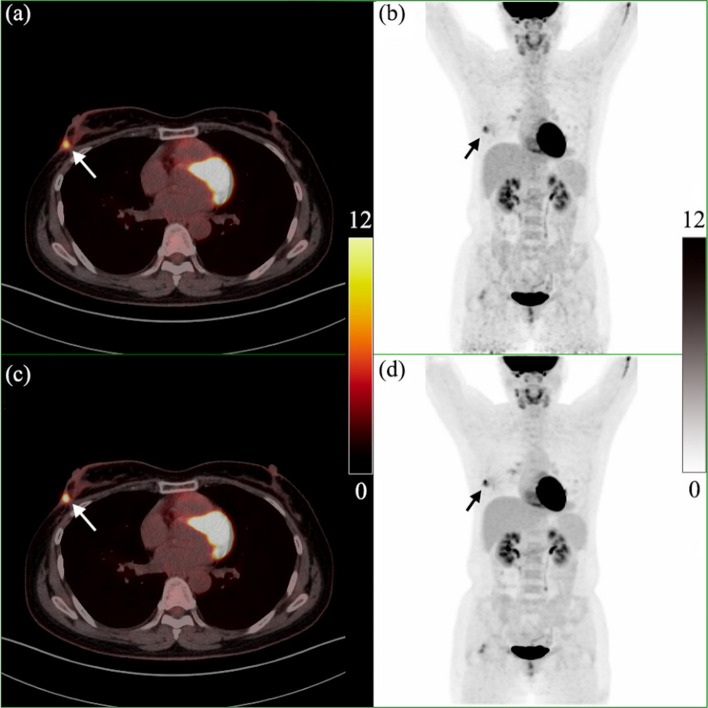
PET/CT image of a breast cancer patient. a and c are PET/CT fusion images reconstructed by OSEM and DPL, respectively; b and d are MIPs reconstructed by OSEM and DPL, respectively. A recurrence lesion (seen the arrow) after surgery with a maximal diameter of 0.87 cm was found in the left breast. The SUV_max_ of this lesion was 5.4 for OSEM, and 6.5 for DPL
